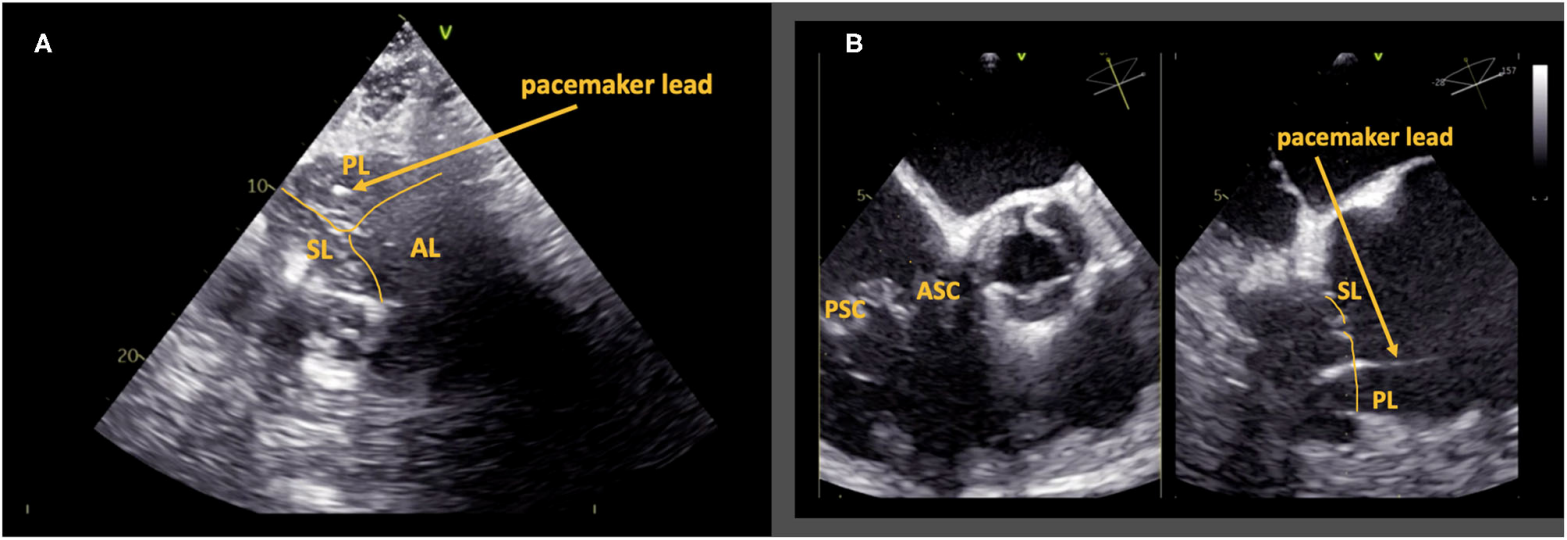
Case 2: Leaflet perforation. Transthoracic en face view of the tricuspid valve shows the pacemaker lead passing through the posterior leaflet (A). In transesophageal echocardiography, a deep-transesophageal commissural view of the tricuspid valve (B, left side) with the corresponding 90° view of the septal and the posterior leaflet (B, right side) proves perforation of the posterior leaflet. AL, anterior leaflet; PL, posterior leaflet; SL, septal leaflet; PSC, postero-septal commissure; ASC, antero-septal commissure.
